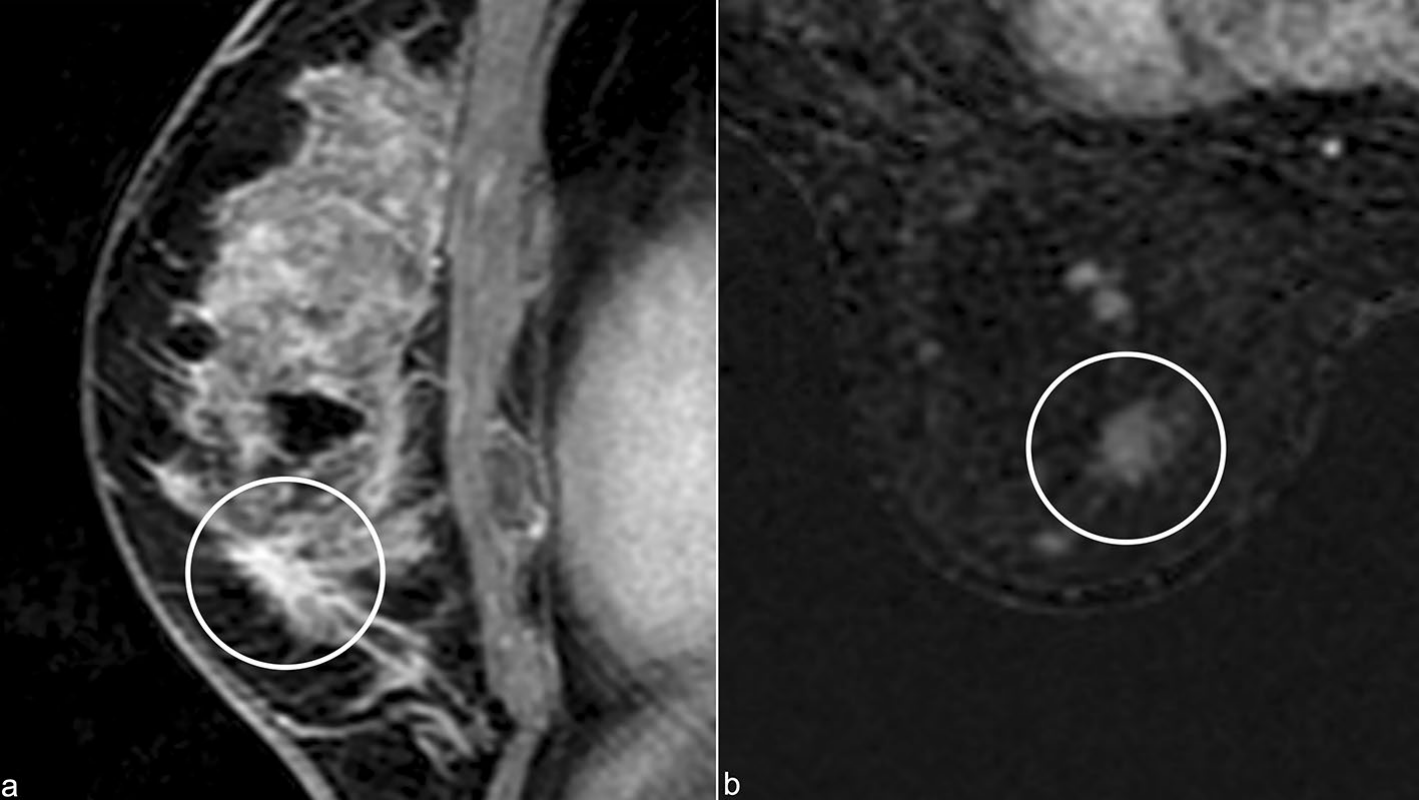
Sagittal MRI contrast material-enhanced T1-weighted image (a) and axial MRI subtracted early contrast-enhanced image (b) show an enhancing mass with irregular borders (white circle)
